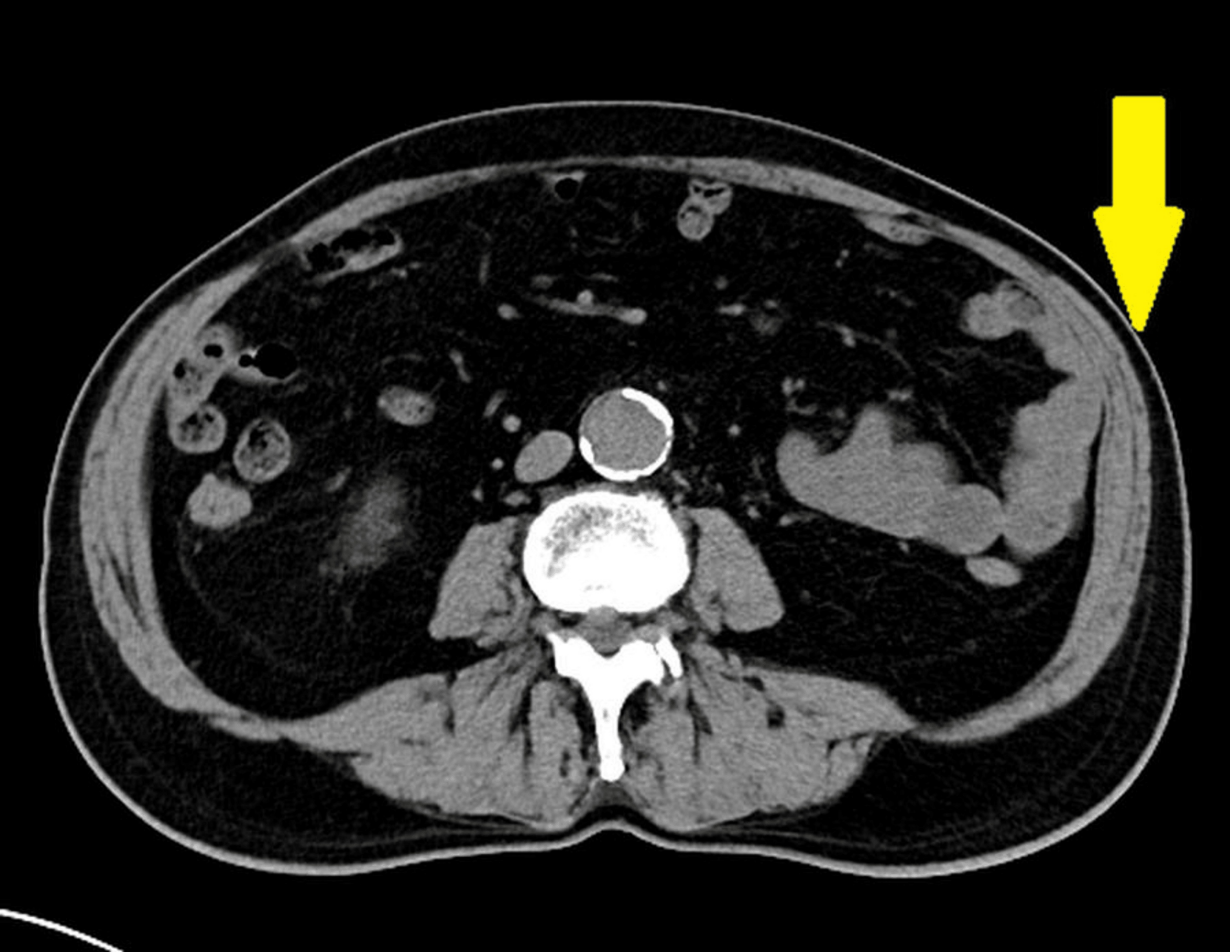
Abdominal muscle thinning on the left side following herpes zoster. CT image showing asymmetric thinning of the left abdominal wall muscles (arrow), consistent with segmental motor paresis following herpes zoster infection. No evidence of true herniation is observed.
